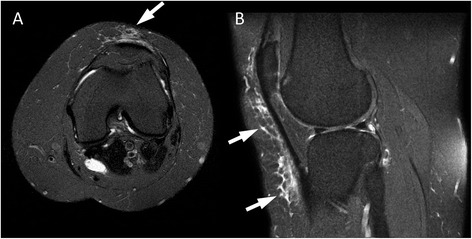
Axial T2-weighted fat-saturated image at the most posterior aspect of the femoral condyles (a), and midline sagittal PD-weighted fat-saturated image (b) from an MRI examination of the knee of a 46 year old female. Arrows demonstrate abnormal subcutaneous T2 hyper-intense signal anterior to the knee extensor mechanism
